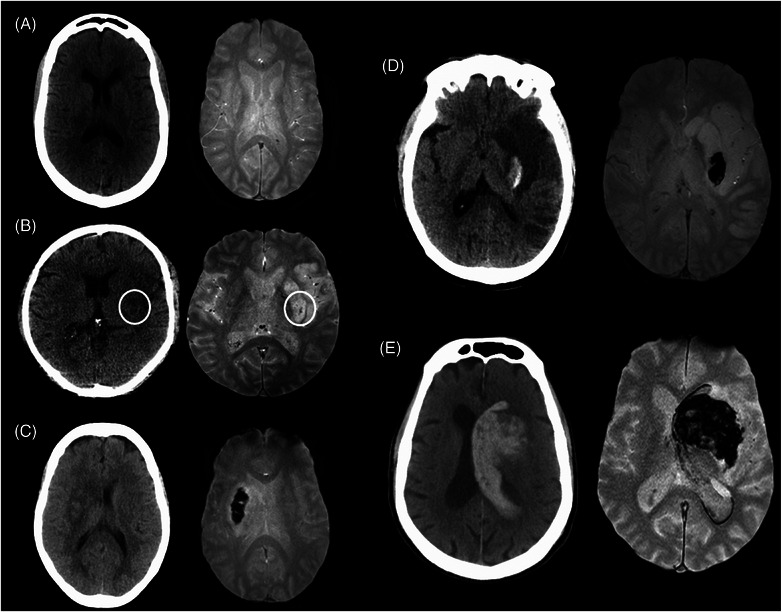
Examples of concordant Heidelberg Bleeding Classification of hemorrhagic transformation between CT and MRI. A, HBC 0: 63‐year‐old female, admission NIHSS score 7, basilar artery (mTICI 2b), CT 7 hours before MRI. B, HBC 1a: 74‐year‐old male, admission NIHSS score 20, intravenous alteplase, M1 MCA (mTICI 3), CT 2 hours before MRI. C, HBC 1b: 61‐year‐old female, admission NIHSS score 15, M1 MCA (mTICI 2b), CT 1 hour after MRI. D, HBC 1c: 73‐year‐old male, admission NIHSS score 23, iICA (mTICI 3), CT 7 hours after MRI. E, HBC 2: 74‐year‐old male, admission NIHSS score 28, iICA (mTICI 2b), CT 6 hours before MRI. CT indicates computed tomography; HBC, Heidelberg Bleeding Classification; iICA, intracranial internal carotid artery; MCA, middle cerebral artery; MRI, magnetic resonance imaging; mTICI, modified Thrombolysis in Cerebral Infarction; and NIHSS, National Institutes of Health Stroke Scale.
